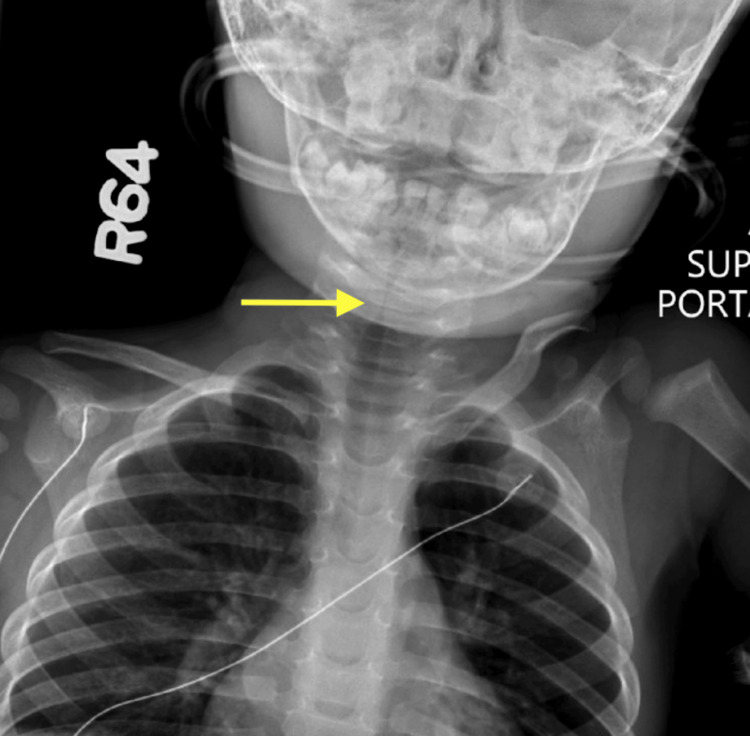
Posterior-anterior radiograph showing initial subglottic stenosis and diffuse tracheal narrowing
